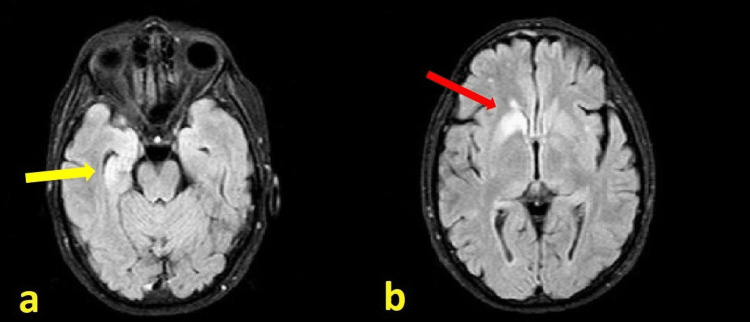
Magnetic resonance imaging of the brain MRI fluid-attenuated inversion recovery (FLAIR) showing hyperintensity in the medial right temporal lobe (a; yellow arrow) and anterior right caudatus (b; red arrow).
